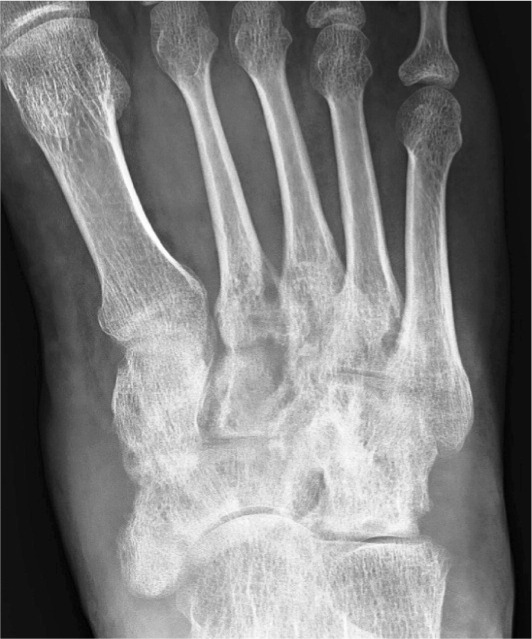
Post operative radiograph of the foot showing healing of the lytic lesions.
